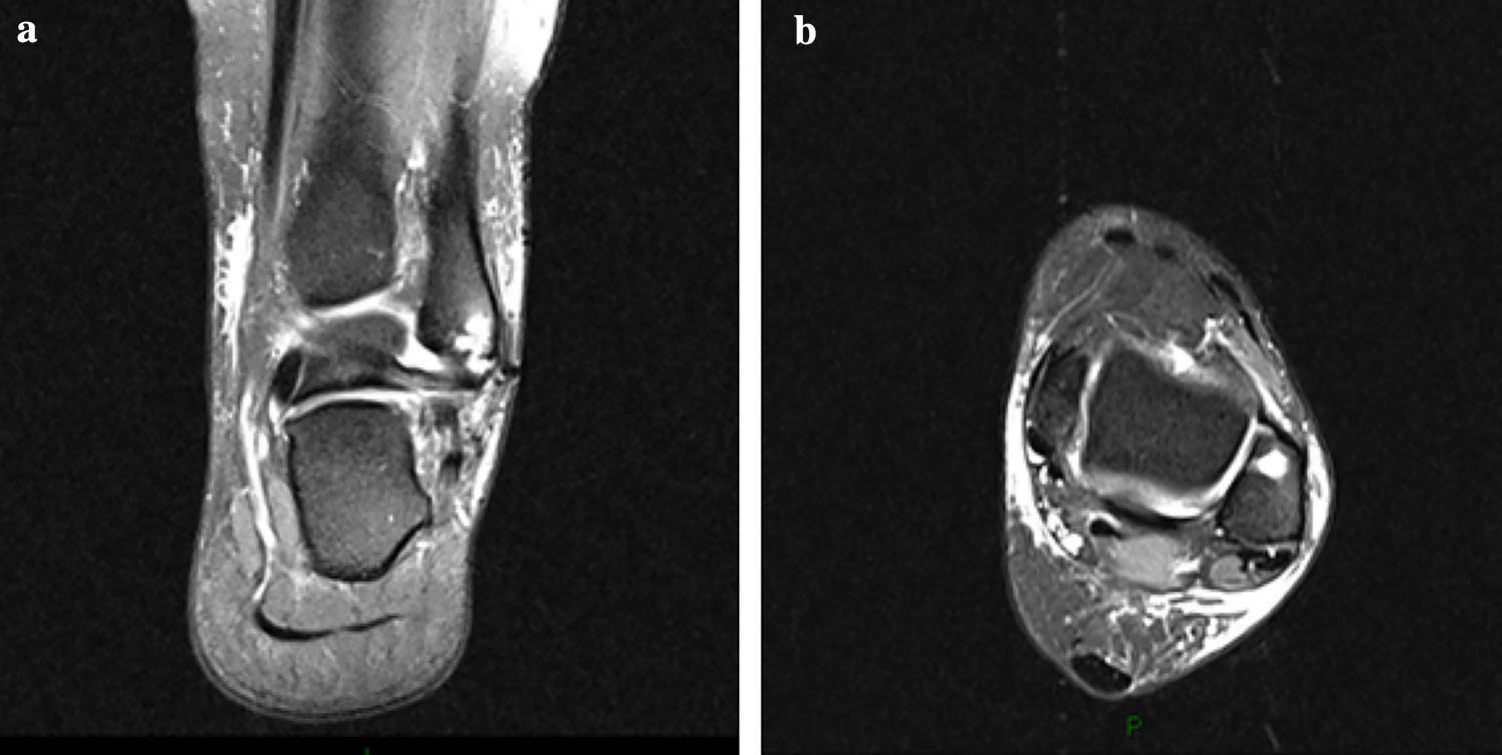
Post-operative MRI scan with anchors (a coronal view, b axial view)
